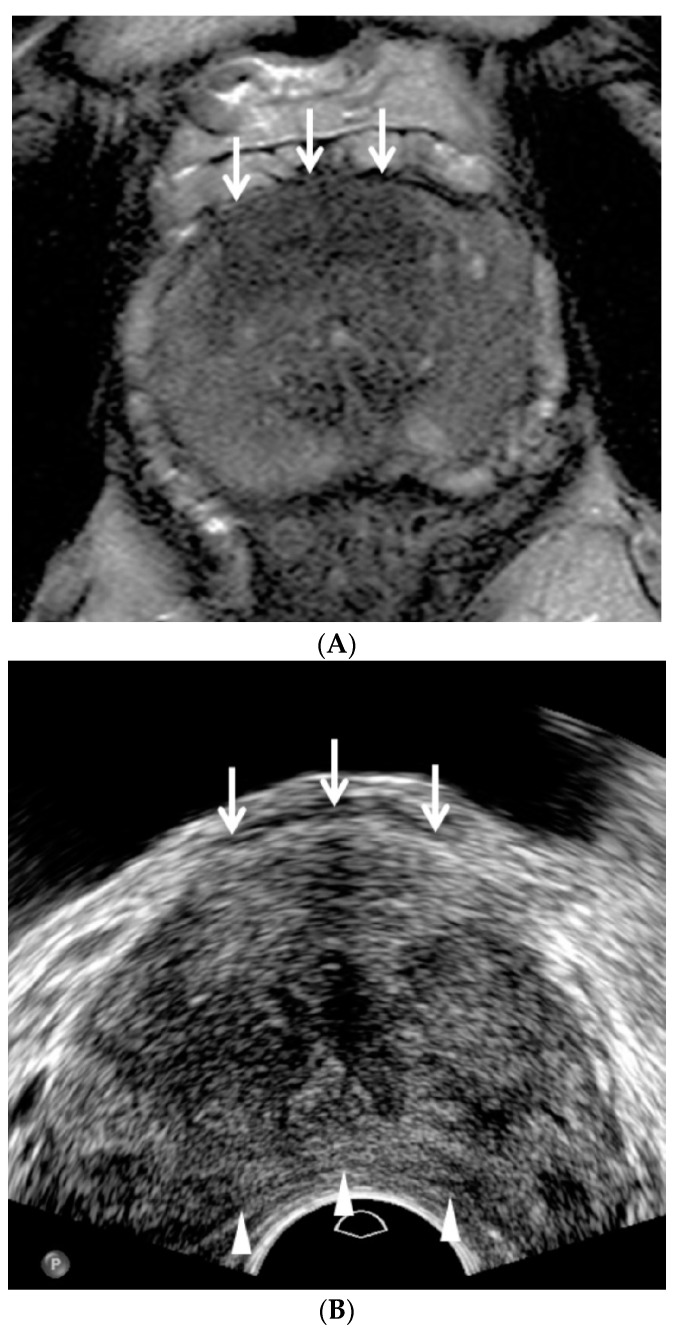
A PI-RADS 5 transition cancer in a 61-year-old man. (A) T2-weighted axial MR image shows a moderately hypointense transition lesion (white arrows) in the anterior midline base measuring 2.7 cm, suggesting that the PI-RADS lesion category is 5. The patient’s PSA level was 12.27 ng/mL prior to biopsy. (B) TRUS axial image clearly shows a hyperechoic transition lesion (white arrows) in the anterior midline mid-gland. The TRUS lesion is located more inferiorly to the MRI lesion. White arrowheads indicate that the prostate is compressed by a TRUS probe to reduce the tumor-to-probe distance. A TRUS-guided targeted biopsy alone was performed with five cores, in which three and one confirmed Gleason scores of 7 (4 + 3) and 6 (3 + 3) adenocarcinomas, respectively.
